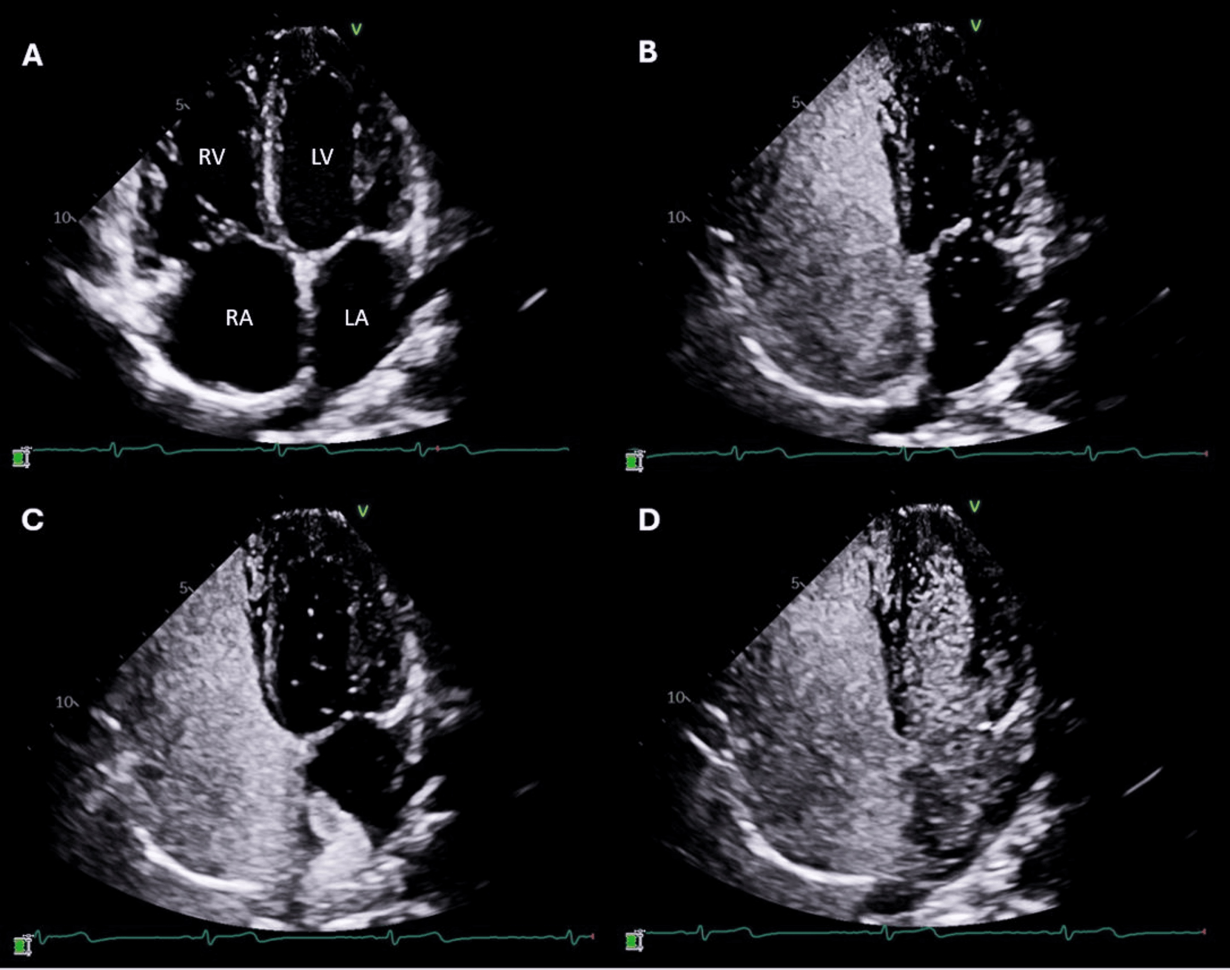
Positive agitated saline contrast study demonstrating a surge of bubbles from the direction of the right pulmonary vein after two cardiac cycles. (A) Apical four-chamber view demonstrating right atrial (RA) and right ventricular (RV) dilation. (B) Agitated saline contrast enters the right-sided chambers, and a few bubbles appear in the left atrium (LA). (C) Surge of bubbles entering the LA from the direction of the right pulmonary vein within two cardiac cycles. (D) Bubbles completely opacify the left-sided chambers, including the left ventricle (LV), by the third cardiac cycle.
